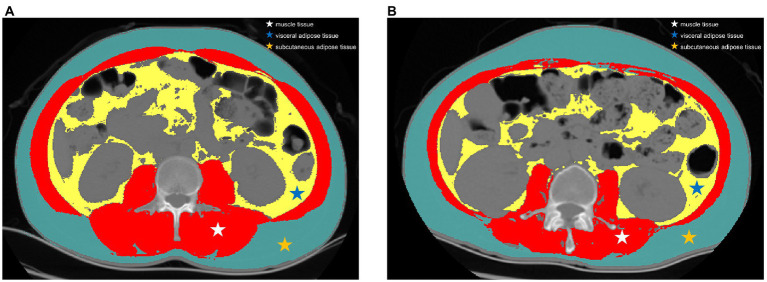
Representative computed tomography scans at the third lumbar vertebra level in patients with and without sarcopenia. (A) a patient with high skeletal muscle index and (B) a patient with low skeletal muscle index. Red region: skeletal muscle mass, assessed using thresholds of-29 to 150 Hounsfield units; yellow region: visceral adipose tissue, assessed using thresholds of-150 to-50 Hounsfield units; green region: subcutaneous adipose tissue, assessed using thresholds of −190 to −30 Hounsfield units.
